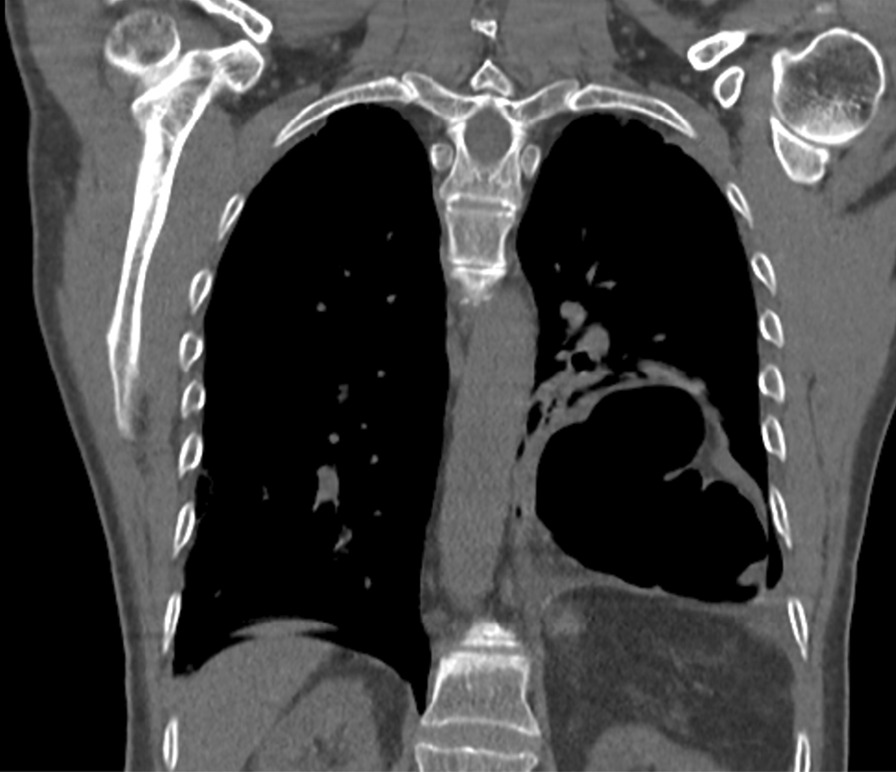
The colon which herniated into the thorax is shown in coronal view of the thoracoabdominal computed tomography scan of the patient, performed at the time of their last admission. (The colon was shown with a red arrow, and fluid was shown with an arrowhead)
